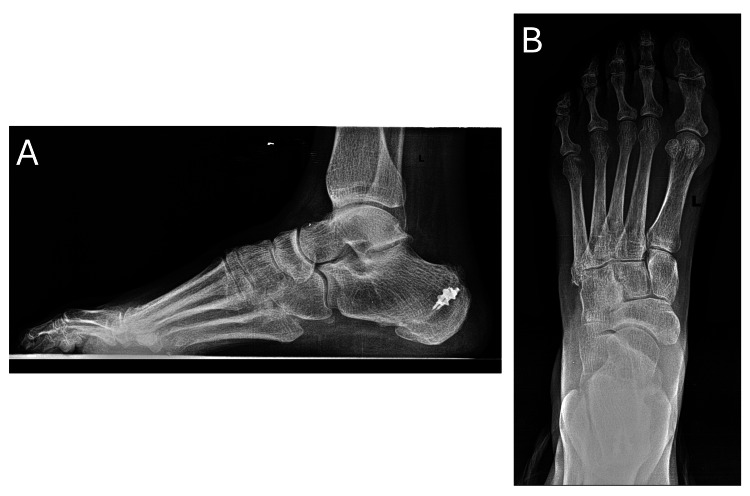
Radiographs prior to PTT transfer Pre-procedure left foot radiographs (lateral and AP) performed in October 2022. (A) Lateral view shows two anchors from prior retrocalcaneal exostectomy with detachment and reattachment of the Achilles tendon. (B) AP view remains unchanged from the initial encounter. PTT, posterior tibial tendon
